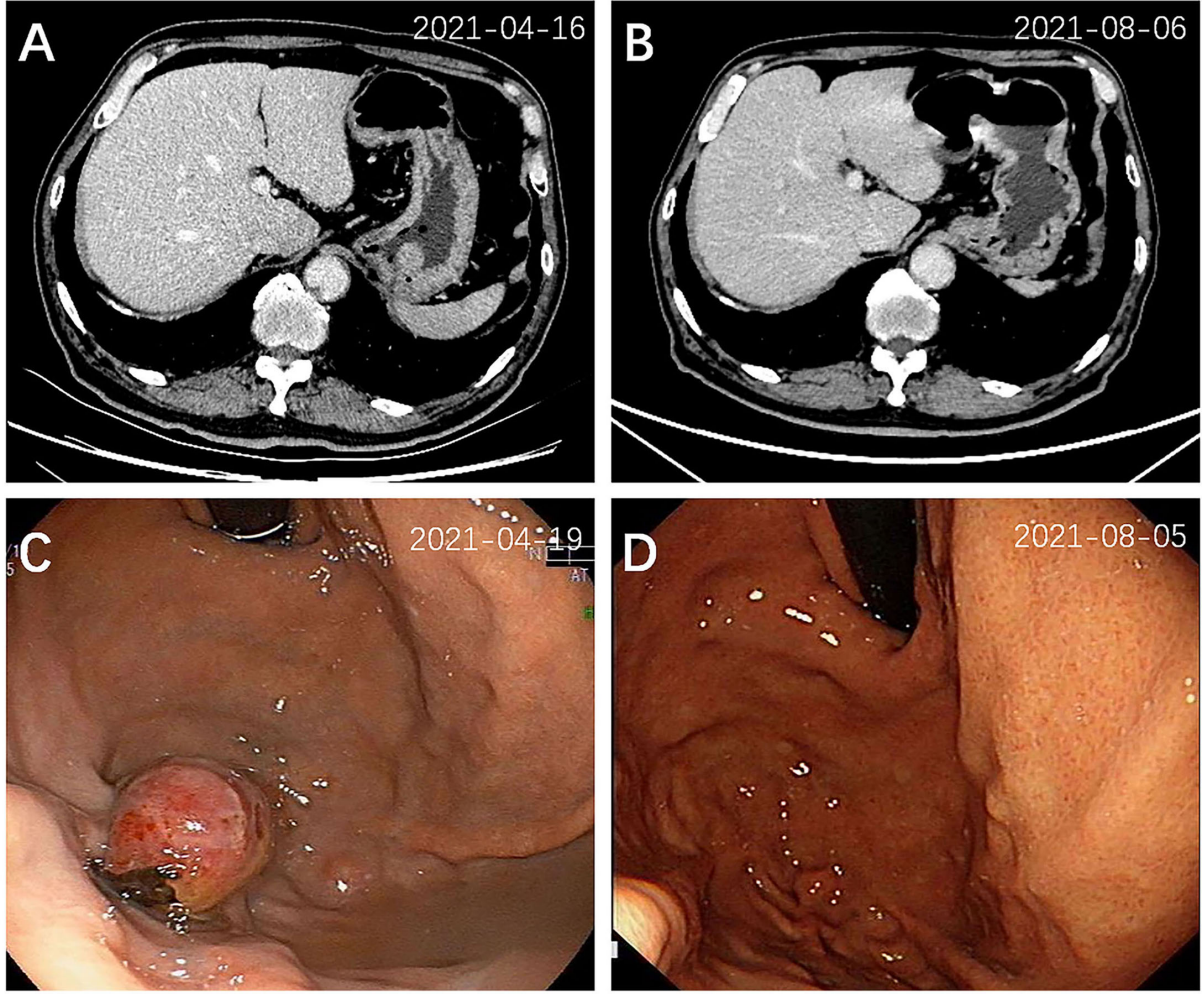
Abdominal CT scan and endoscopic view of the gastric tumor at diagnosis (A, C) and 3 months after treatment (B, D). A mass (2.0 cm × 2.0 cm) located in the gastric fundus was detected by CT (A) and gastroscopy (C), which disappeared 3 months after treatment (B, D).
